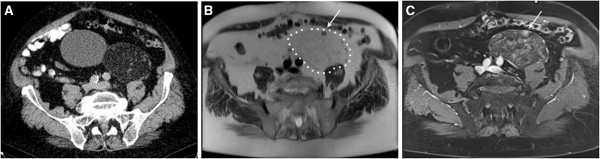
Computed tomography (A) and magnetic resonance imaging findings (B and C) demonstrate an ovoid encapsulated retroperitoneal mass, which contained predominantly fat and a few solid hypervascularized nodules (arrows).
